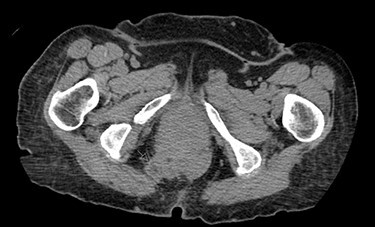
Post-operative CT demonstrating mild fluid in pelvis and resolution of the hernia (black arrow).
